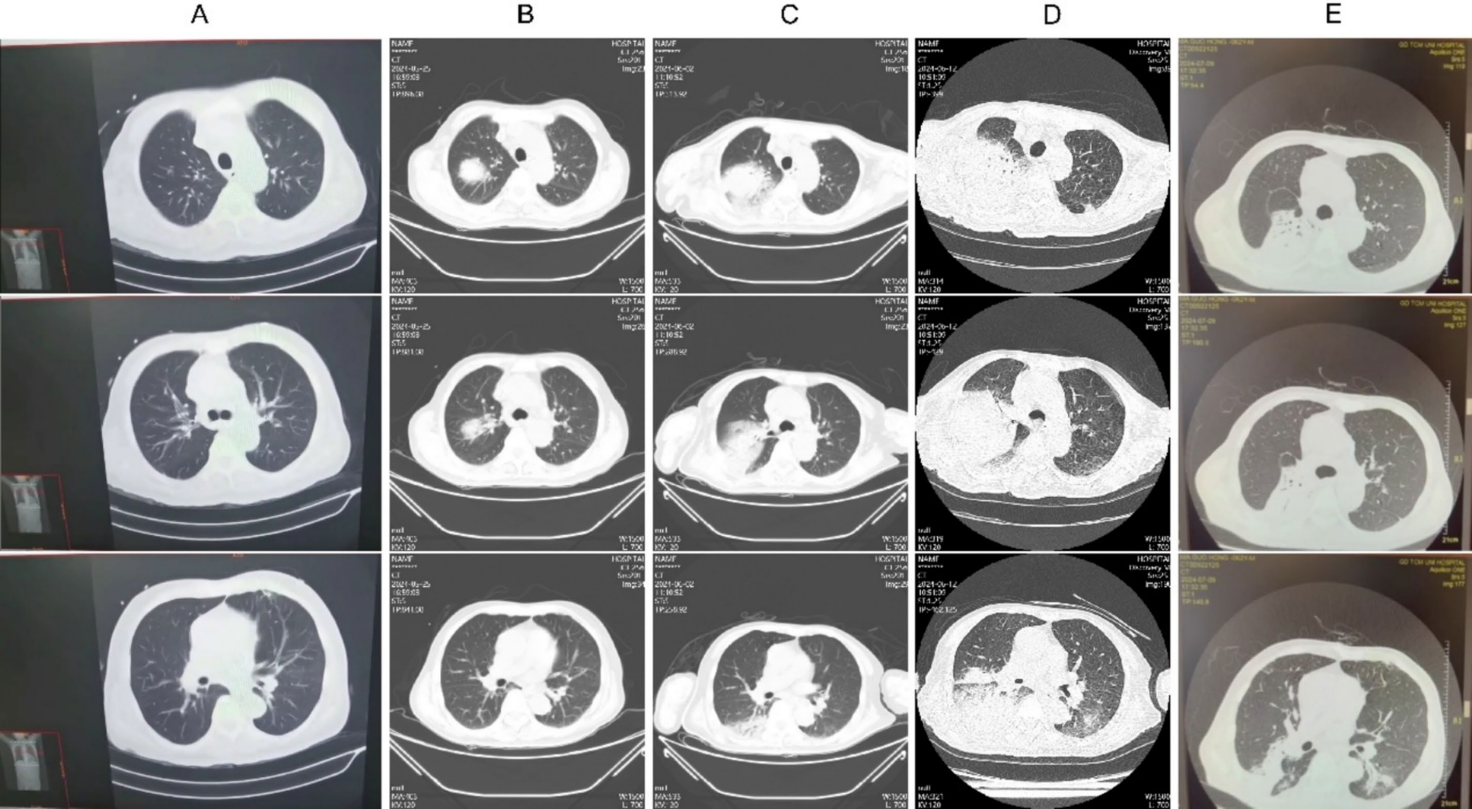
Chest CT imaging alterations during the patient’s clinical management. (A) May 14, 2014; (B) May 25, w2024; (C) June 2, 2024; (D) June 12, 2024; (E) July 9, 2024.
